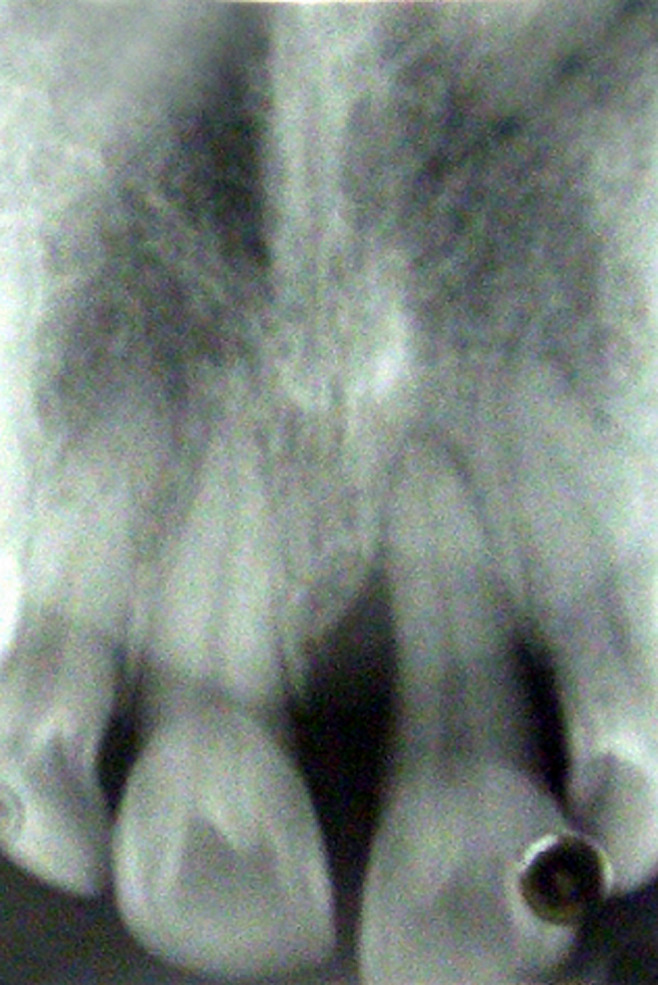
X-ray shows periapical radiolucency
